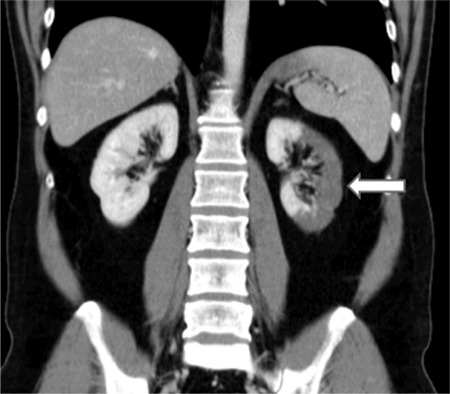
Abdominal computed tomography with contrast showing a hypodense area in the left kidney involving the anterolateral component of the upper and middle zones and the entire lower pole.
